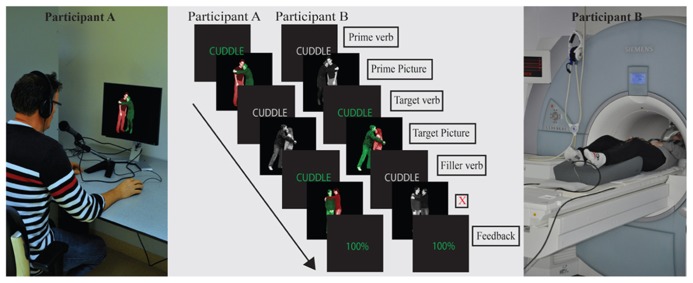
Set-up of the experiment in the communicative context condition. Two participants – one in the MRI scanner, one in the behavioral experiment room – describe photographs to each other. (In the non-communicative context, there was only one – MRI – participant.) Trial structure and task were identical in both conditions. Green verbs at the start of a trial indicated that a (color-coded) production photograph would follow; gray verbs indicated a (grayscale) comprehension photograph would follow. Verbs were presented to participants in Dutch (English translation is shown in the figure). Production photographs were color-coded to guide participants’ production: participants were instructed to name the green figure before the red figure, leading them to produce an active or a passive sentence. When participant A in the communicative context produces a description, participant B listens to the description, and *vice versa*. Mismatches in the communicative context were created by showing a different photograph to speaker and listener (in the non-communicative context, a non-matching sentence recording was played to the participant). In both contexts, the listener needs to press a button when a mismatch is noticed. Feedback screens were only present in the communicative context: they reflect the percentage of hits minus false alarms and misses by both participants. Feedback was only presented within a filler block.
